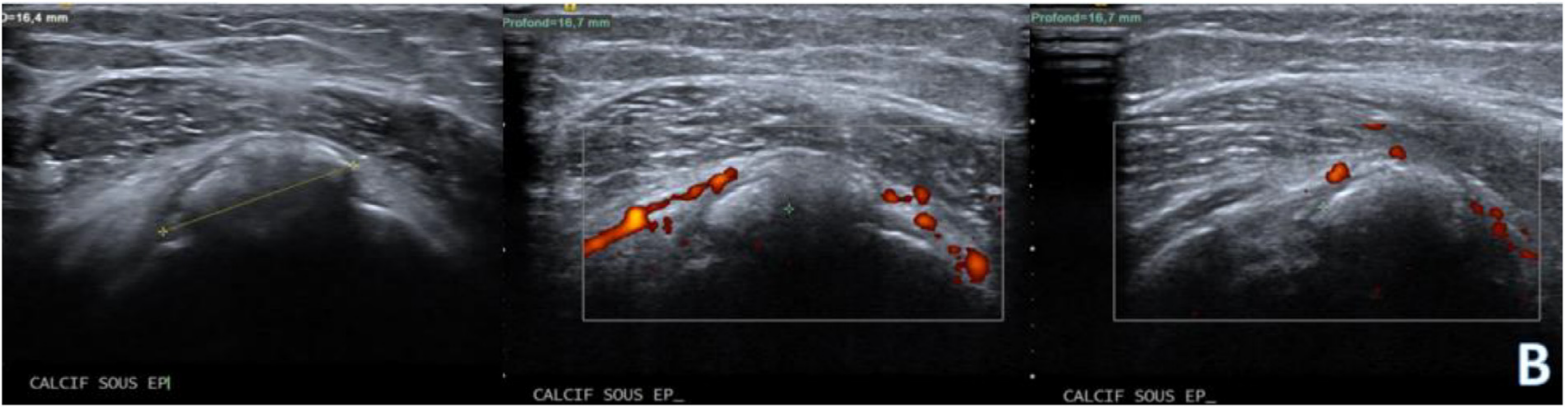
Shoulder ultrasound mode B; hyperechoic foci with acoustic shadowing in the infraspinatus tendon with increased vascularity within the infraspinatus muscle belly extending distally towards the teres minor muscle.
